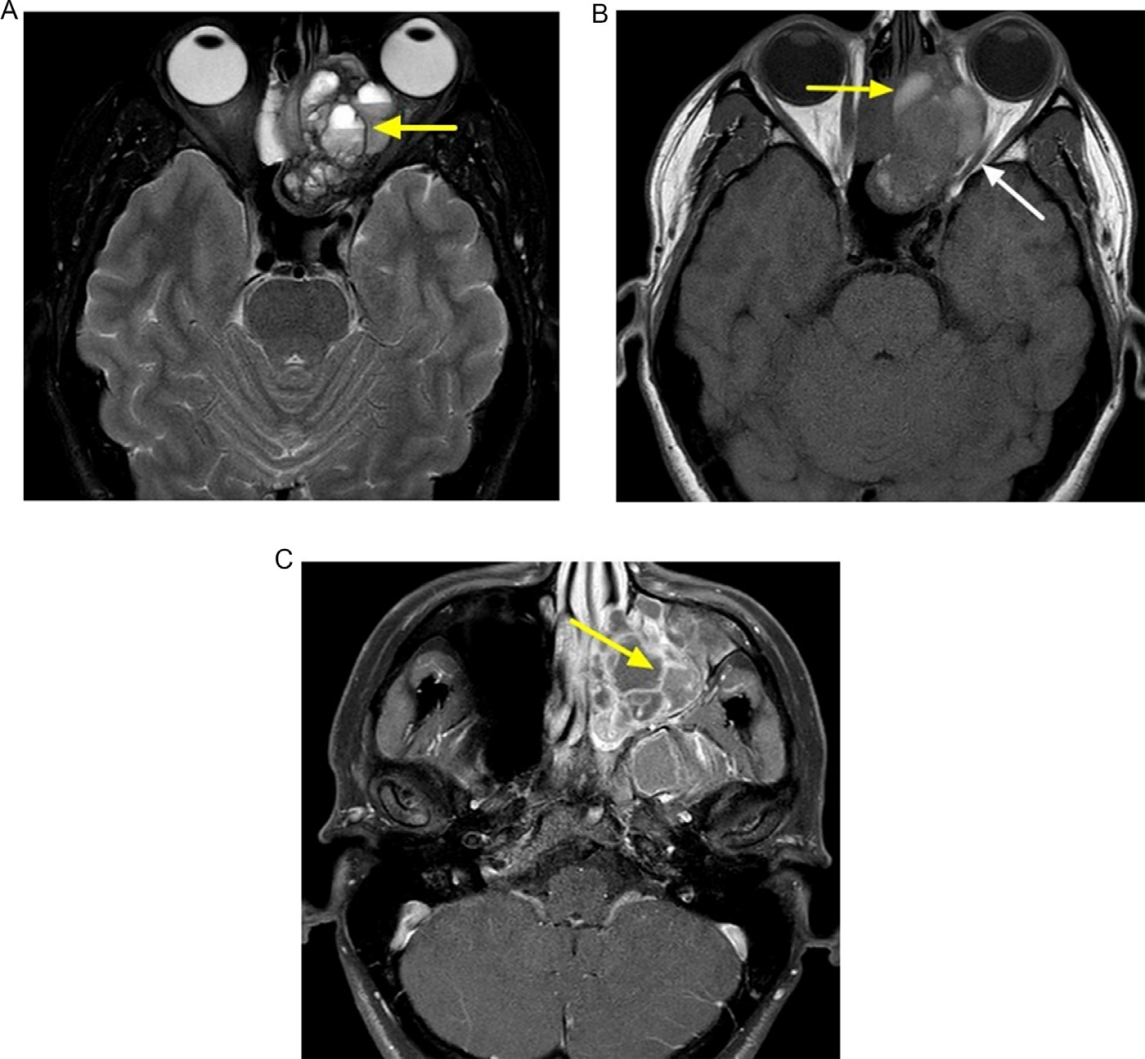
Skull base MRI. (A) Axial T2 confirms multiple cysts with fluid-fluid levels within the nasal cavity mass (yellow arrow). (B) Axial T1 precontrast demonstrates areas of intrinsic T1 hyperintensity consistent with blood products (yellow arrow) and note is made of mass effect on the adjacent left optic nerve (white arrow). (C) Axial T1 postcontrast shows numerous thin, enhancing septations (yellow arrow), without focal enhancing soft tissue component. (Color version of figure is available online.)
